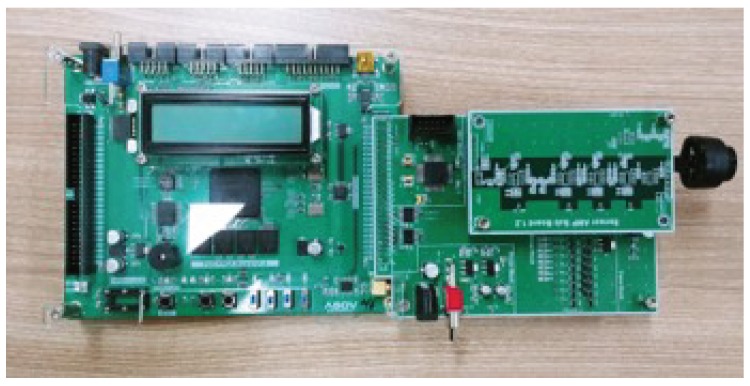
Implemented ultrasonic modem.
